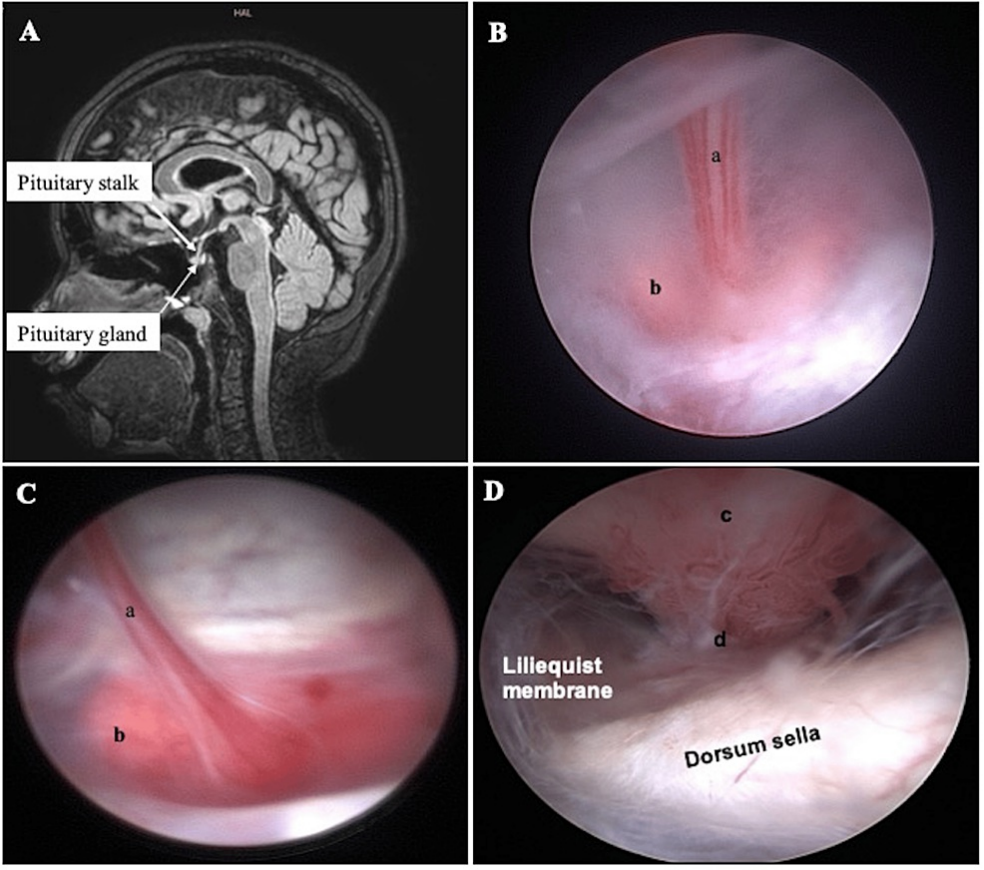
The pituitary stalk and gland. (A) Sagittal T1-weighted brain MRI shows the pituitary stalk and gland that occupies the sella. (B, C) In the endoscopic view, the pituitary stalk is covered with fenestrated capillary loops, and the reddish-grey gland has a richer, smaller vessel (plexus). (D) The dorsum sella is visualized posteriorly, and the area is laterally covered with a Liliequist membrane. a, pituitary stalk; b, pituitary gland; c, tuber cinereum; d, the junction between the stalk and the gland as identified by the beginning of the rich smaller vessel (plexus).
